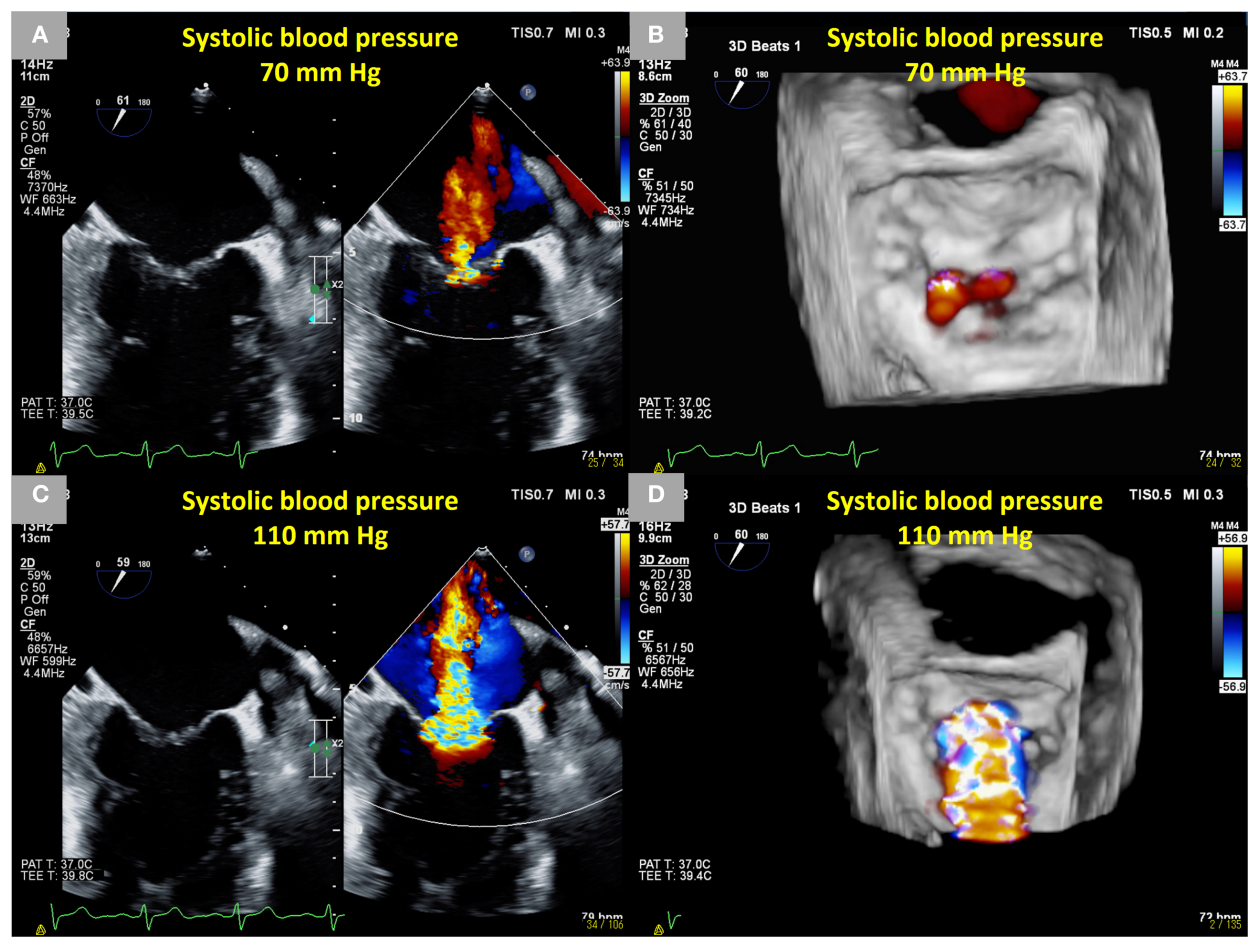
Dynamic changes in SMR during transesophageal echocardiography in a 66-year-old male patient who had an anterior old myocardial infarction and heart failure with reduced ejection fraction. Mild SMR (EROA 0.10 cm^2^) under sedation using midazolam under a systolic blood pressure of approximately 70 mm Hg. (A) Two-dimensional B-mode and color Doppler images from the intercommissural view and (B) a three-dimensional color Doppler image from the en-face view. Dynamic severe SMR (EROA 0.51 cm^2^) under an elevated systolic blood pressure of approximately 110 mm Hg using norepinephrine. (C) Two-dimensional B-mode and color Doppler images from the intercommissural view and (D) a three-dimensional color Doppler image from the en-face view. SMR, secondary mitral regurgitation; EROA, effective regurgitant orifice area.
